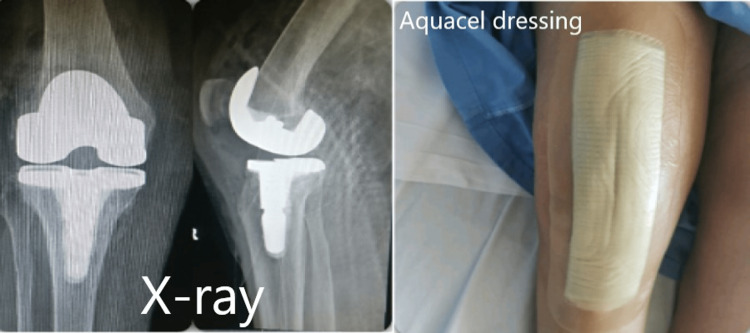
X-ray of knee replacement and Aquacel dressing
